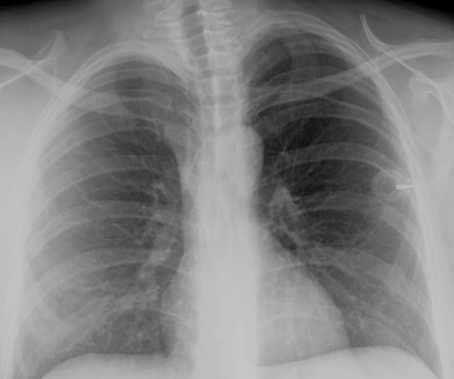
Postoperative x-ray after catheter implantation. The catheter tip is placed correctly in the superior caval vein.
